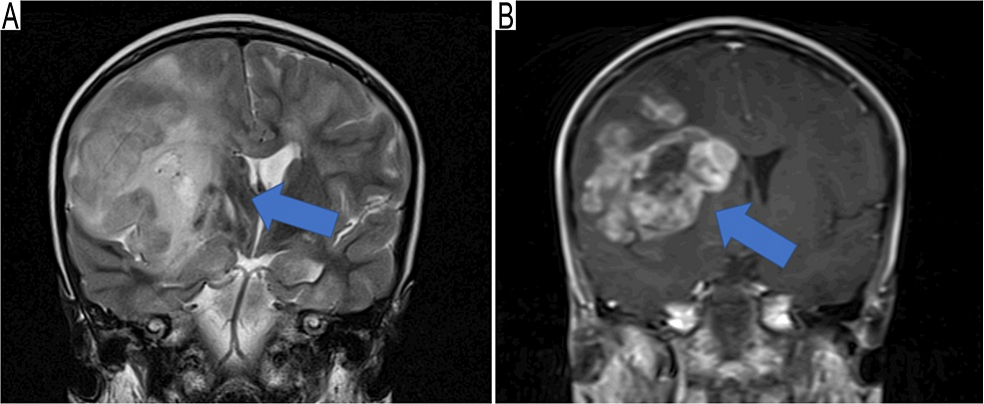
Imaging studies A predominantly right frontal mass. (A) T2-weighted image shows a heterogeneous high signal intensity (arrow) with minor surrounding edema. (B) T1-weighted image with contrast shows a heterogeneous pattern of enhancement (arrow).
